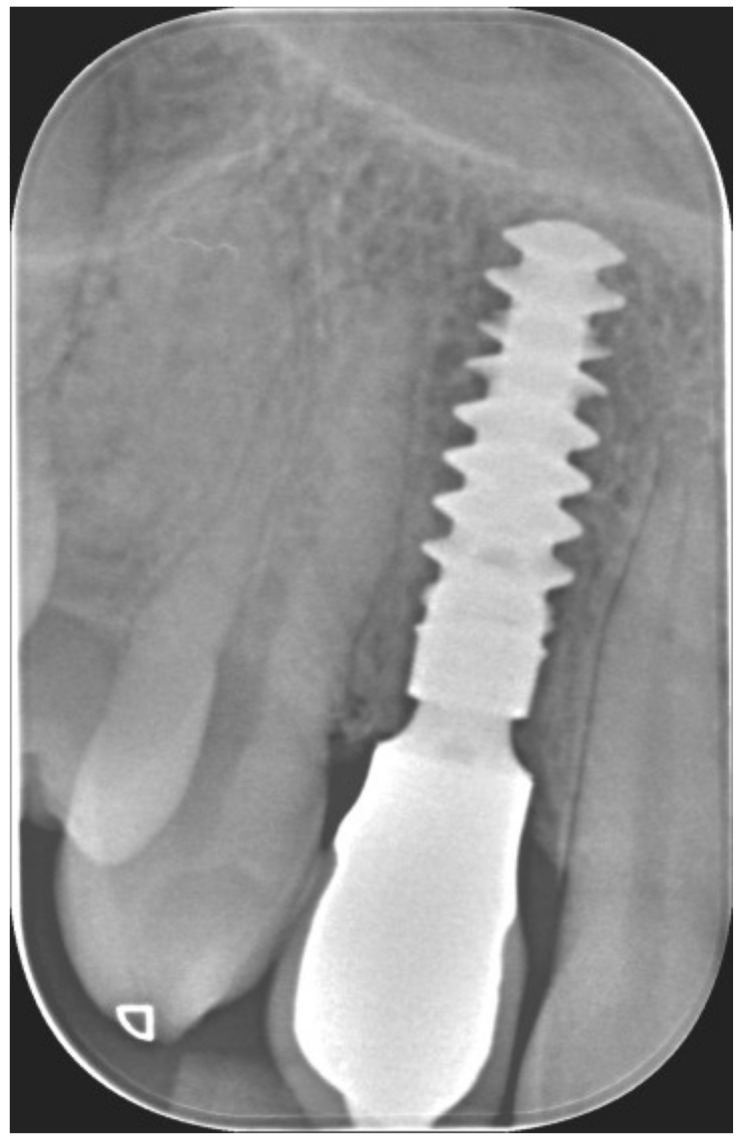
Periapical X-ray at 1 year of follow-up.
